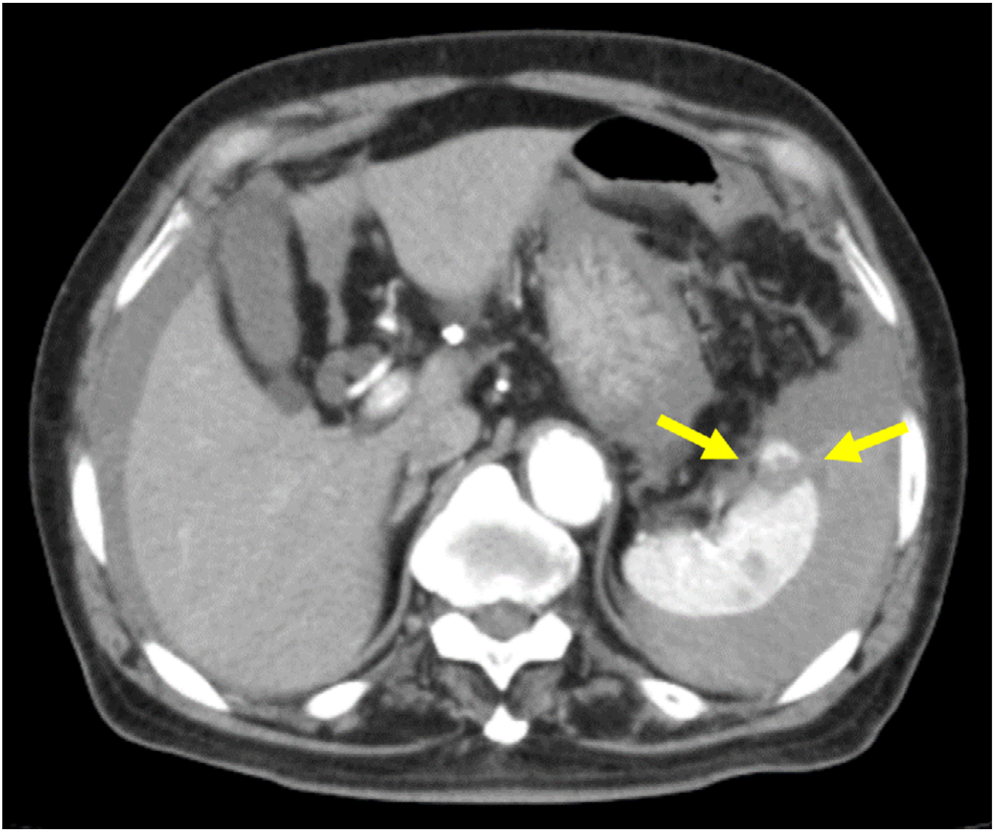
The contrast‐enhanced abdominal computed tomography on the next day. The contrast‐enhanced abdominal computed tomography revealed splenic injury; ascites in the perihepatic, perisplenic, and pelvic area; and low‐density area of the part of the spleen (arrow) without rupture of the splenic artery or extravasation.
